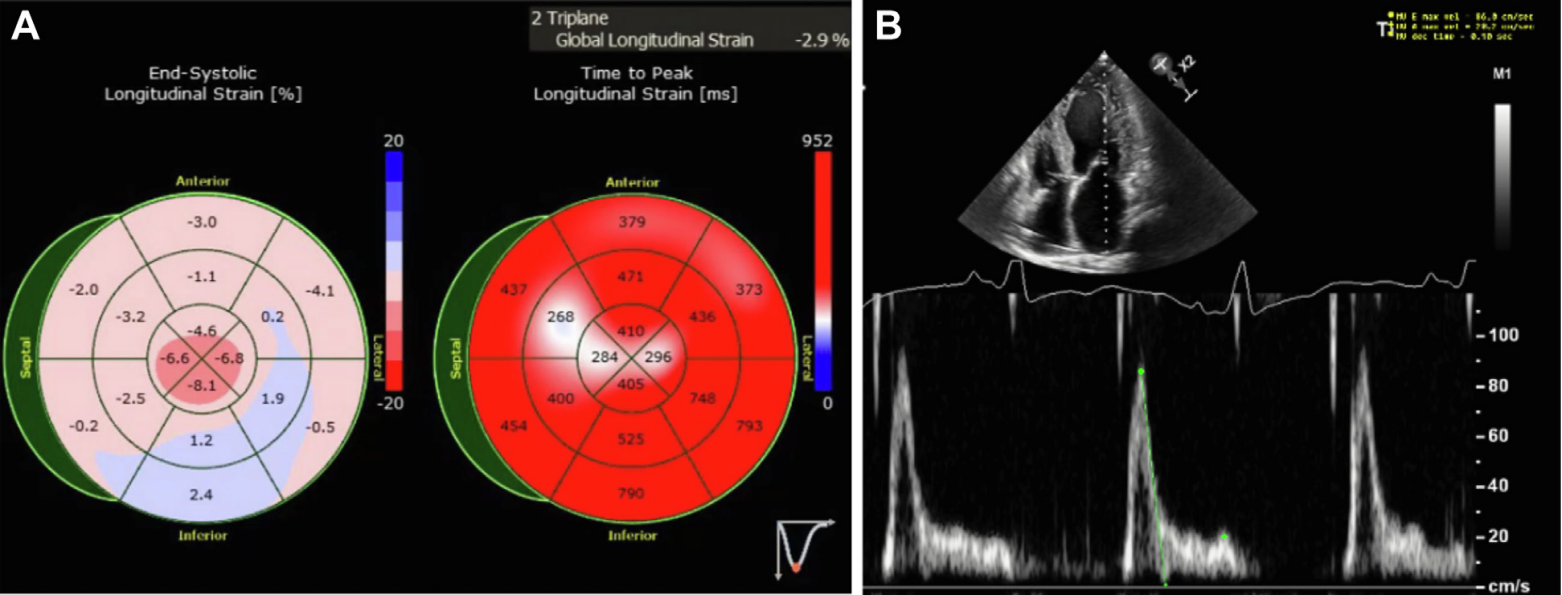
Echocardiogram Images for Patient 3 (A) Patient 3 had reduced global longitudinal strain with apical sparing pattern on transthoracic echocardiogram. (B) Mitral inflow Doppler signal consistent with restrictive filling pattern for patient 3.
